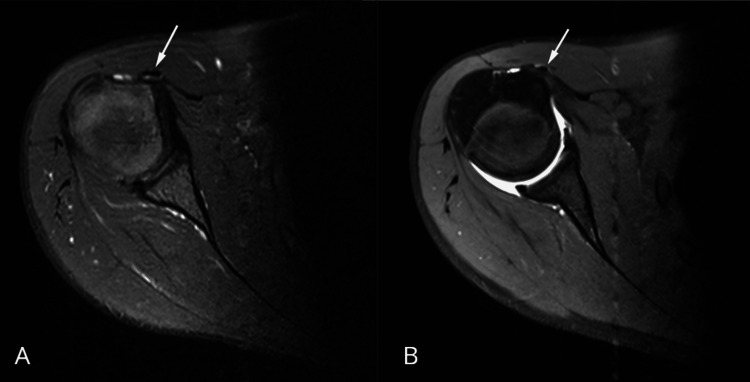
Axial MRI images of the right shoulder A: proton density fat-suppressed sequence demonstrating medial displacement of the long head of the biceps tendon outside the bicipital groove (arrow). B: axial T1-weighted fat-suppressed MR arthrography image with intra-articular contrast confirming extra-articular medial dislocation of the long head of the biceps tendon (arrow)
